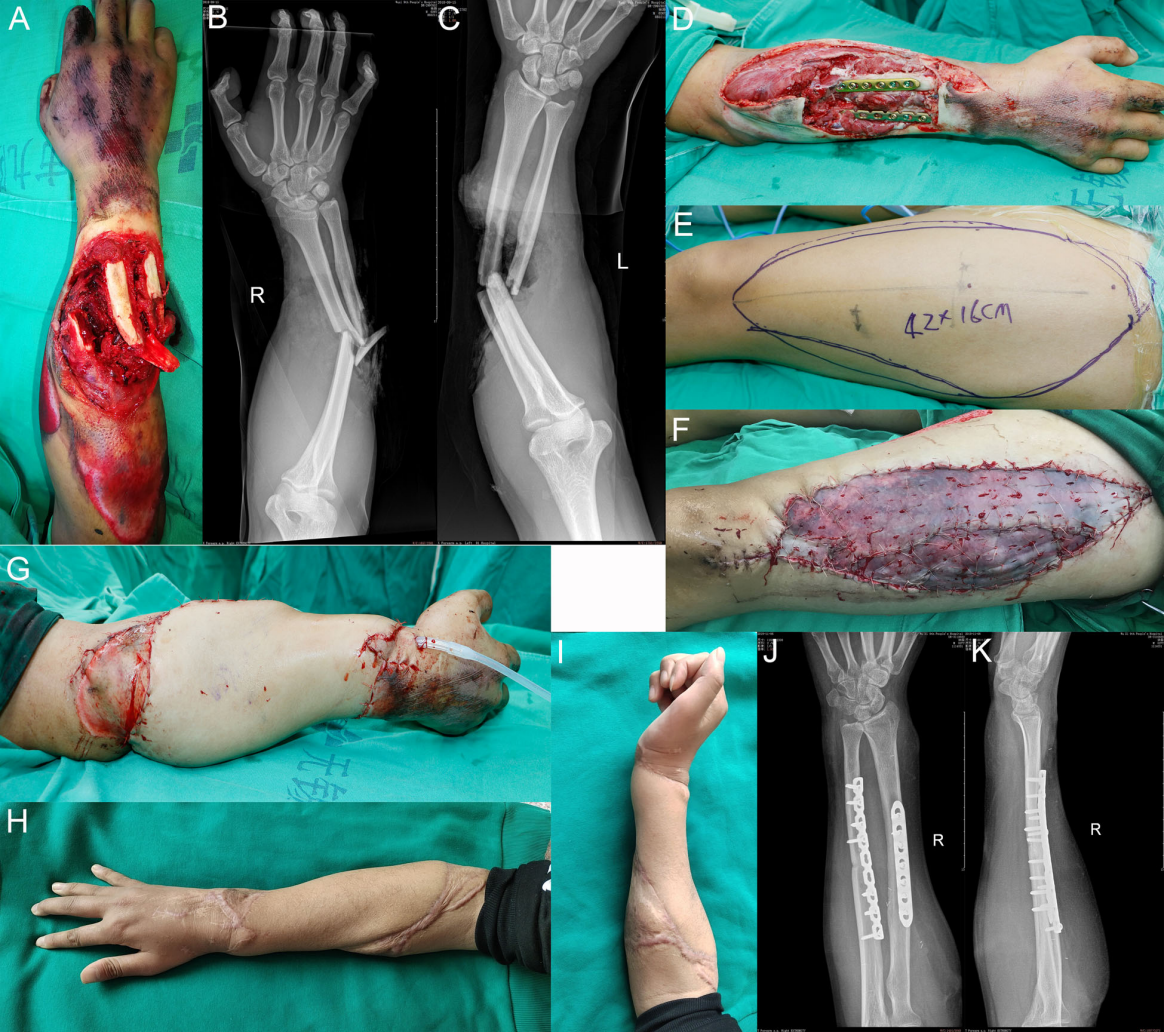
Case 2, a 23-year-old man. a Right arm crush injury with soft tissue defect with cortical defect in the dorsal part of ulna and radius. b, c Both ulnar and radial fractures. d The defect on the right was on the forearm with the size 16 × 42 cm^2^. e–g Flap covering the reconstructed soft tissue. h–i Appearance at 18-month follow-up. j–k The X-ray showed fracture healing at 15 months after surgery.
